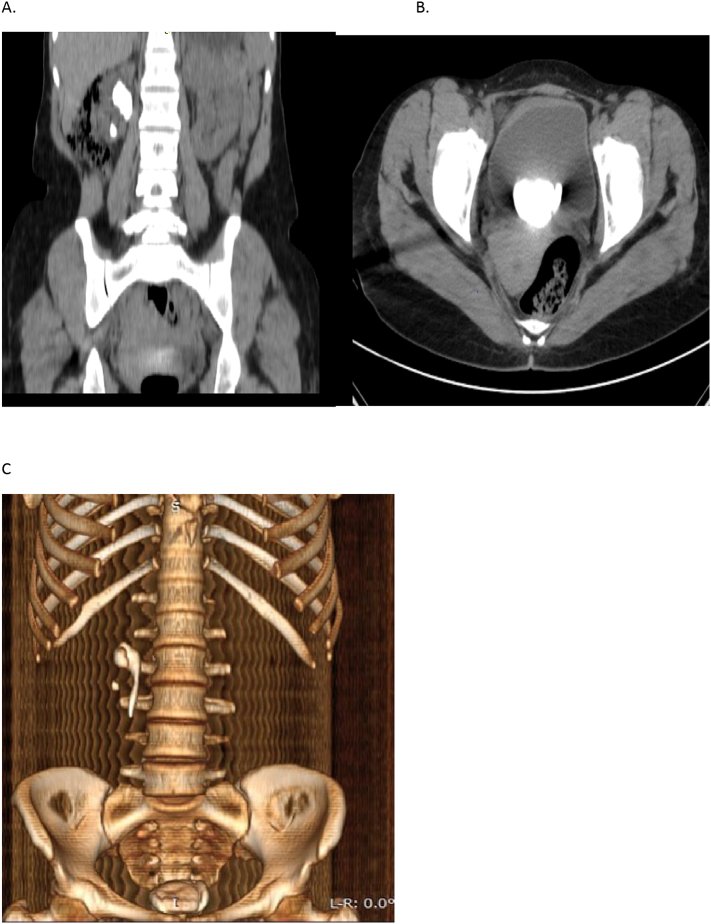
CT scan imaging slices; (A) coronal image showing rt pelvic stone and lower pole stone, (B) an axial image showing huge bladder stone, (C) a 3D reconstruction to show both bladder and rt pelvic stone.
